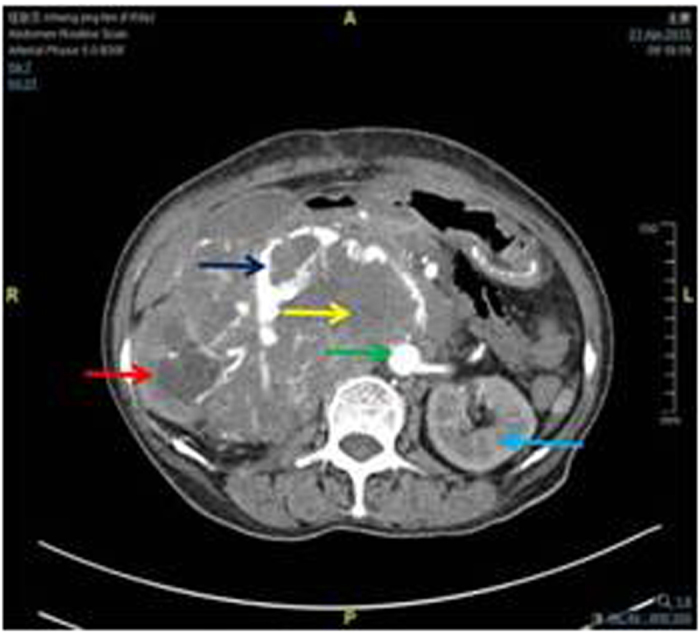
CT imaging of a patient with PRT. In this case, the tumor (yellow arrow) had invaded the inferior vena cava (level II, dark blue arrow), right kidney(red arrow) and left renal vein (blue arrow). The aorta abdominalis was highlighted by a green arrow.
